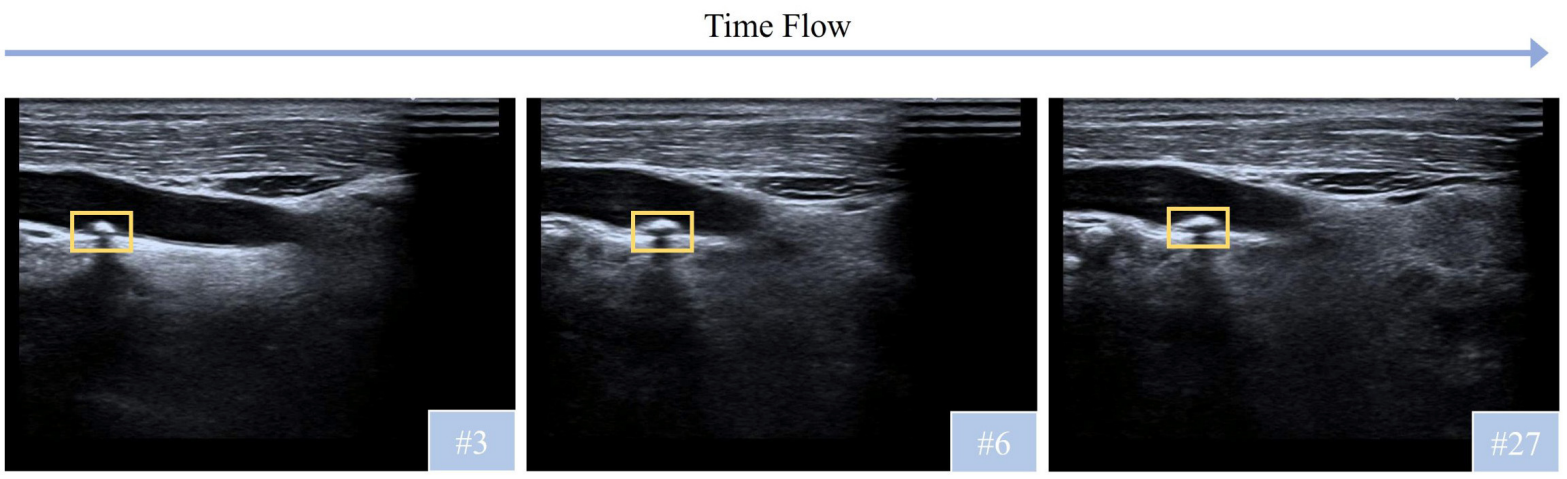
Temporal variation in plaque morphology observed through ultrasound video frames.
